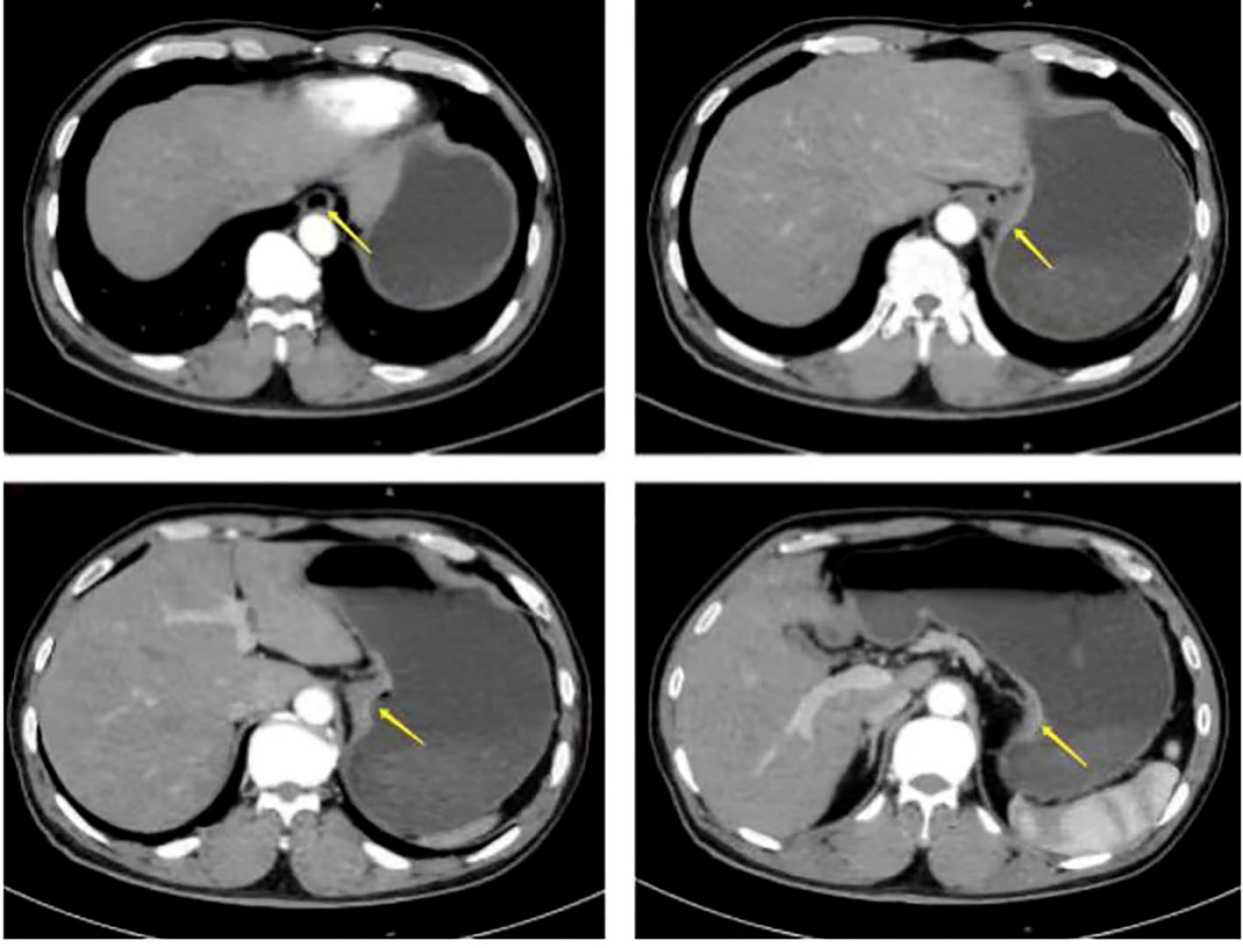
Preoperative computed tomography (CT) scan showed the thickening and enhancement suggestive of malignancy near the gastroesophageal junction but without evidence of regional lymph node involvement or distant spread (arrow).
